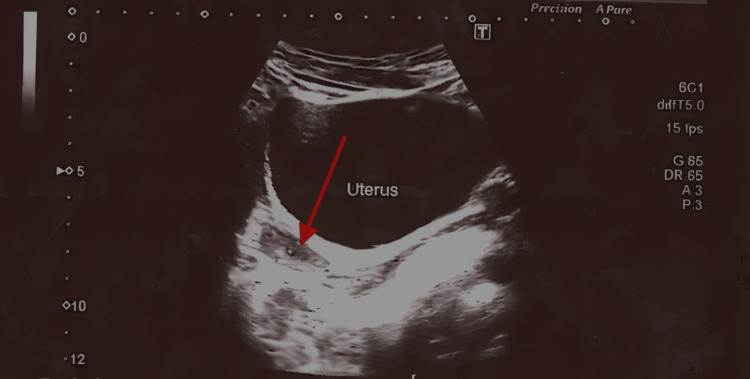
Ultrasound of the pelvis showing an anteverted, small uterus. The red arrow indicates the uterus.
